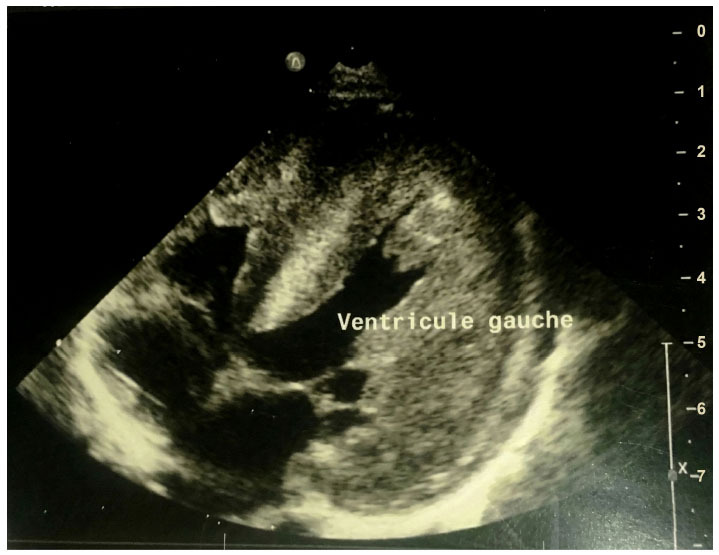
Echocardiography of the LV, parasternal short-axis views. The severe biventricular hypertrophy at diagnosis.
